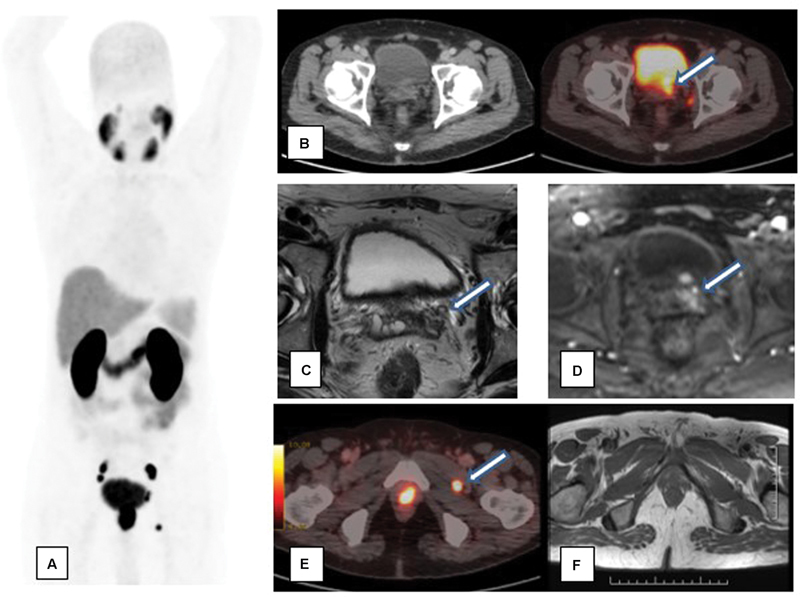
A 72-year-old patient with serum prostate-specific antigen—110 ng/mL, adenocarcinoma prostate with Gleason's score 4 + 5- Concordance between gallium-68 prostate-specific membrane antigen positron emission tomography-computed tomography (Ga-68 PSMA PET/CT) and multiparametric magnetic resonance imaging (mpMRI) in detection of seminal vesicle involvement: Ga-68 PSMA PET/CT showed increased tracer uptake in left seminal vesicle [represented by arrow in axial sections of fusion PET/CT images] (
